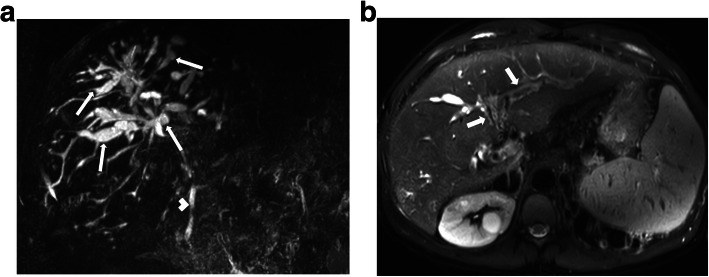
A 30-year-old male patient with known Caroli syndrome presenting with jaundice. a Coronal plane MRCP MIP (maximum intensity projection) image shows multifocal segmental dilation of the intrahepatic bile ducts (arrows). Choledoc is normal (arrowhead). b Axial plane T2W fat-saturated MR image shows increased T2 signal in the periportal area (arrows) and surface irregularity of the liver. These findings are consistent with congenital hepatic fibrosis
